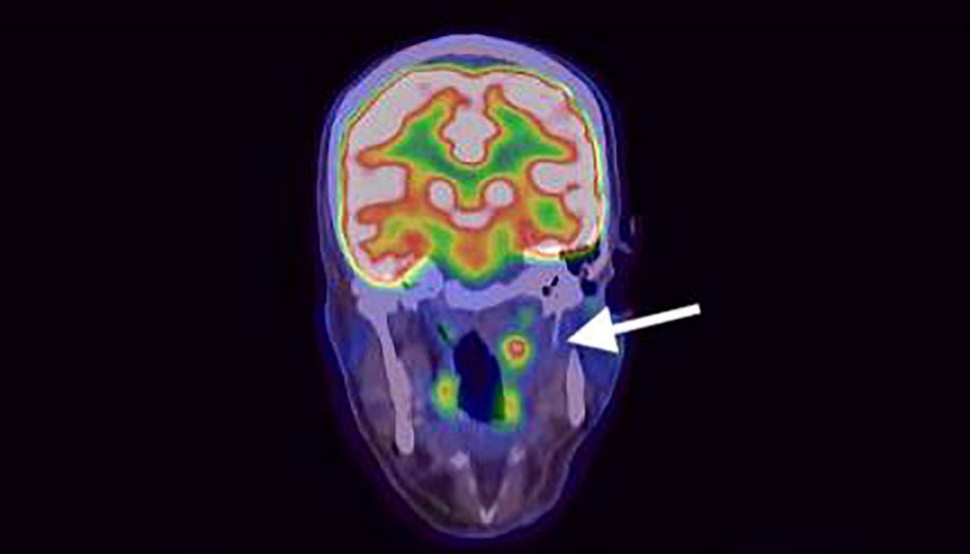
PET/CT with FDG positive mass of the left cranial parapharyngeal space
